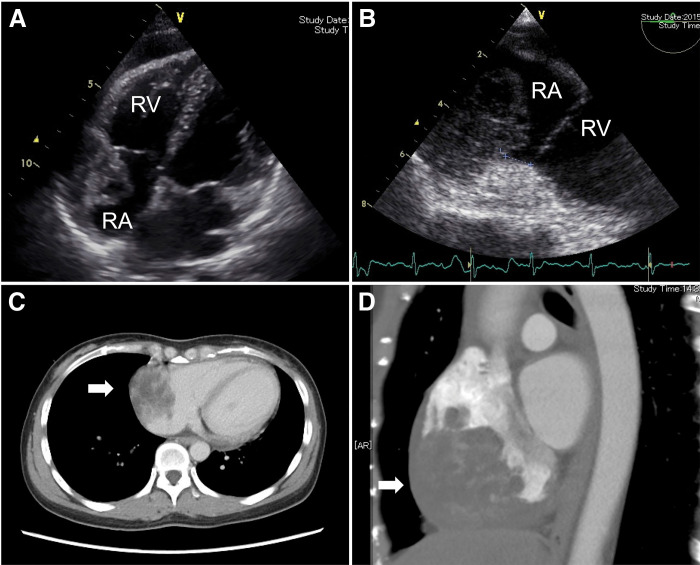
Preoperative imaging findings. (A) TTE demonstrating a large mass occupying the right atrium, accompanied by pericardial effusion. (B) Transesophageal echocardiography revealing a 55 × 41-mm non-pedunculated mass with heterogeneous internal echogenicity, arising from the anterior and superior right atrial wall without tricuspid valve involvement. (C, D) Contrast-enhanced CT showing an irregularly contoured and heterogeneously enhanced mass measuring 54 mm in maximal diameter along the free wall of the right atrium (arrows). RA, right atrium; RV, right ventricle; TTE, transthoracic echocardiography
